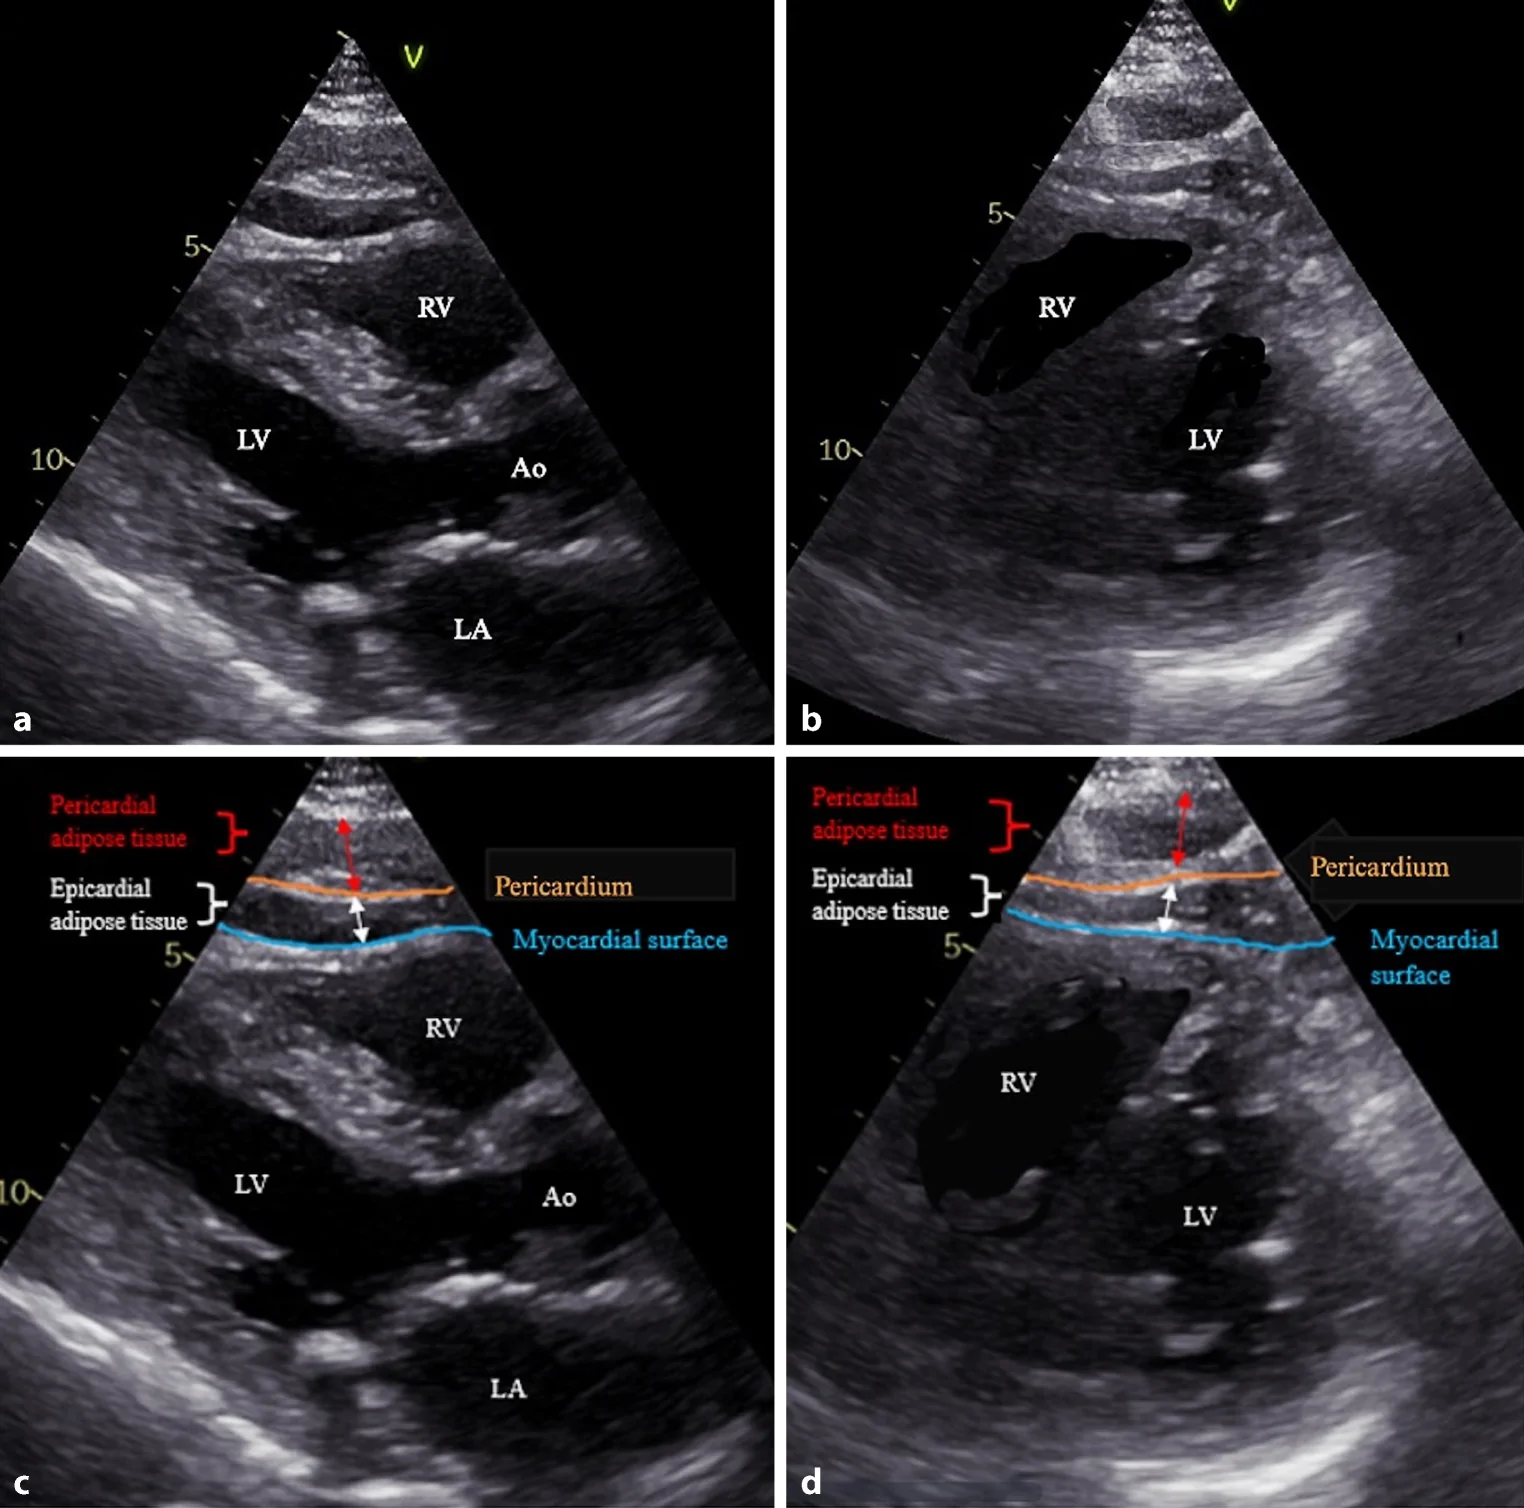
Location of epicardial- and pericardial tissue depots and echocardiographic assessment. a, b Show the parasternal long-axis and short-axis views on the echocardiogram. c, d Show the different layers of adipose tissue (i.e. EAT and PAT) surrounding the myocardium. *White*: EAT, *Red*: PAT. Using the aortic annulus and interventricular septum as reference points. The *orange line* indicates the pericardium, and *blue line* indicates the myocardial surface.* Ao* Aorta;* EAT* epicardial adipose tissue;* PAT* pericardial adipose tissue;* LA* left atrium;* LV* left ventricle;* RV* right ventricle
